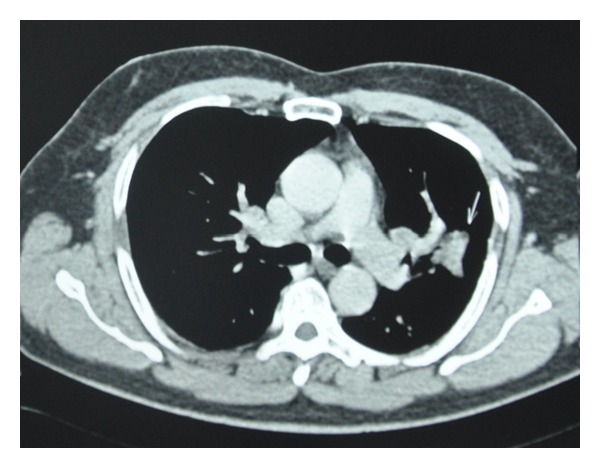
CT scan of the lung (transverse section) showing well-defined nodular lesion measuring 4.1∗3.6 cms in the left upper lobe with spiky margins.
